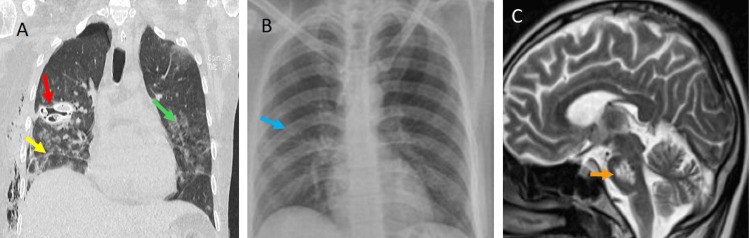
(A) CT scan of the chest shows cavitated lesions (red arrow), cisuritis (yellow arrow), and interstitial infiltrate (green arrow). (B) Three-month follow-up X-ray image with complete recovery (blue arrow). (C) Partial decrement of size lesion in the pons after three months of treatment (orange arrow).
